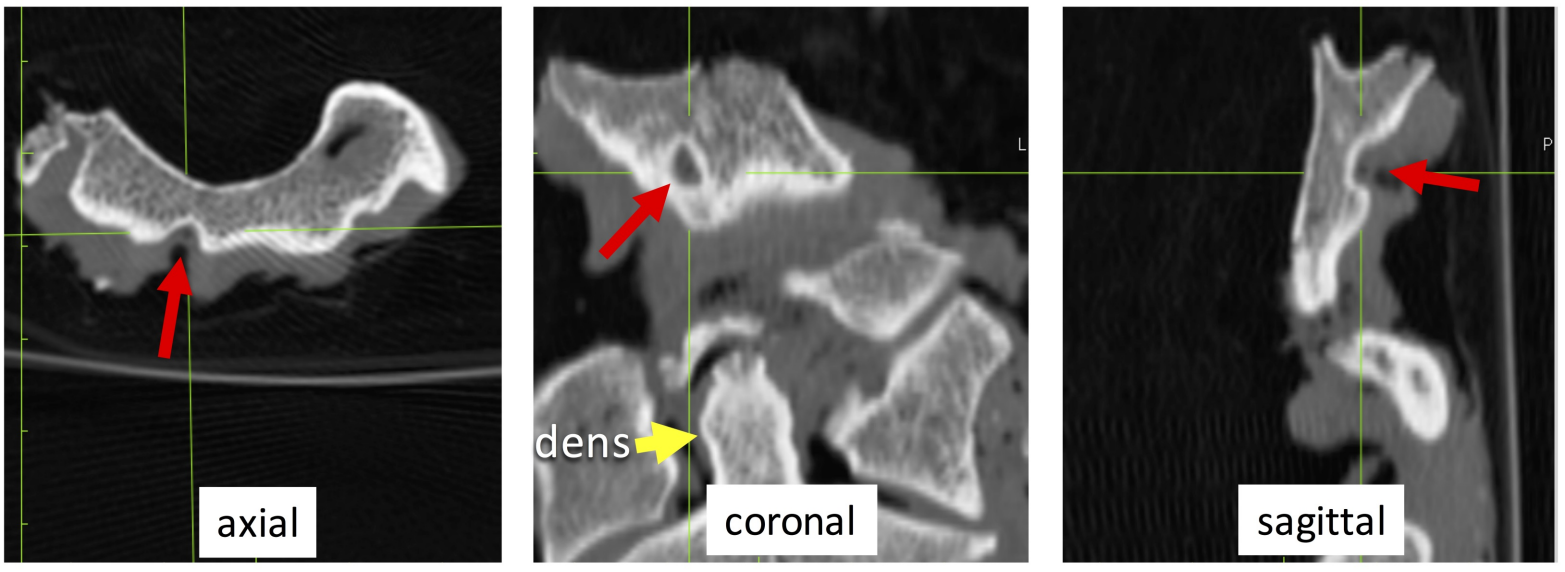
Cross section computed tomography (CT) of the fossa navicularis (red arrow)
